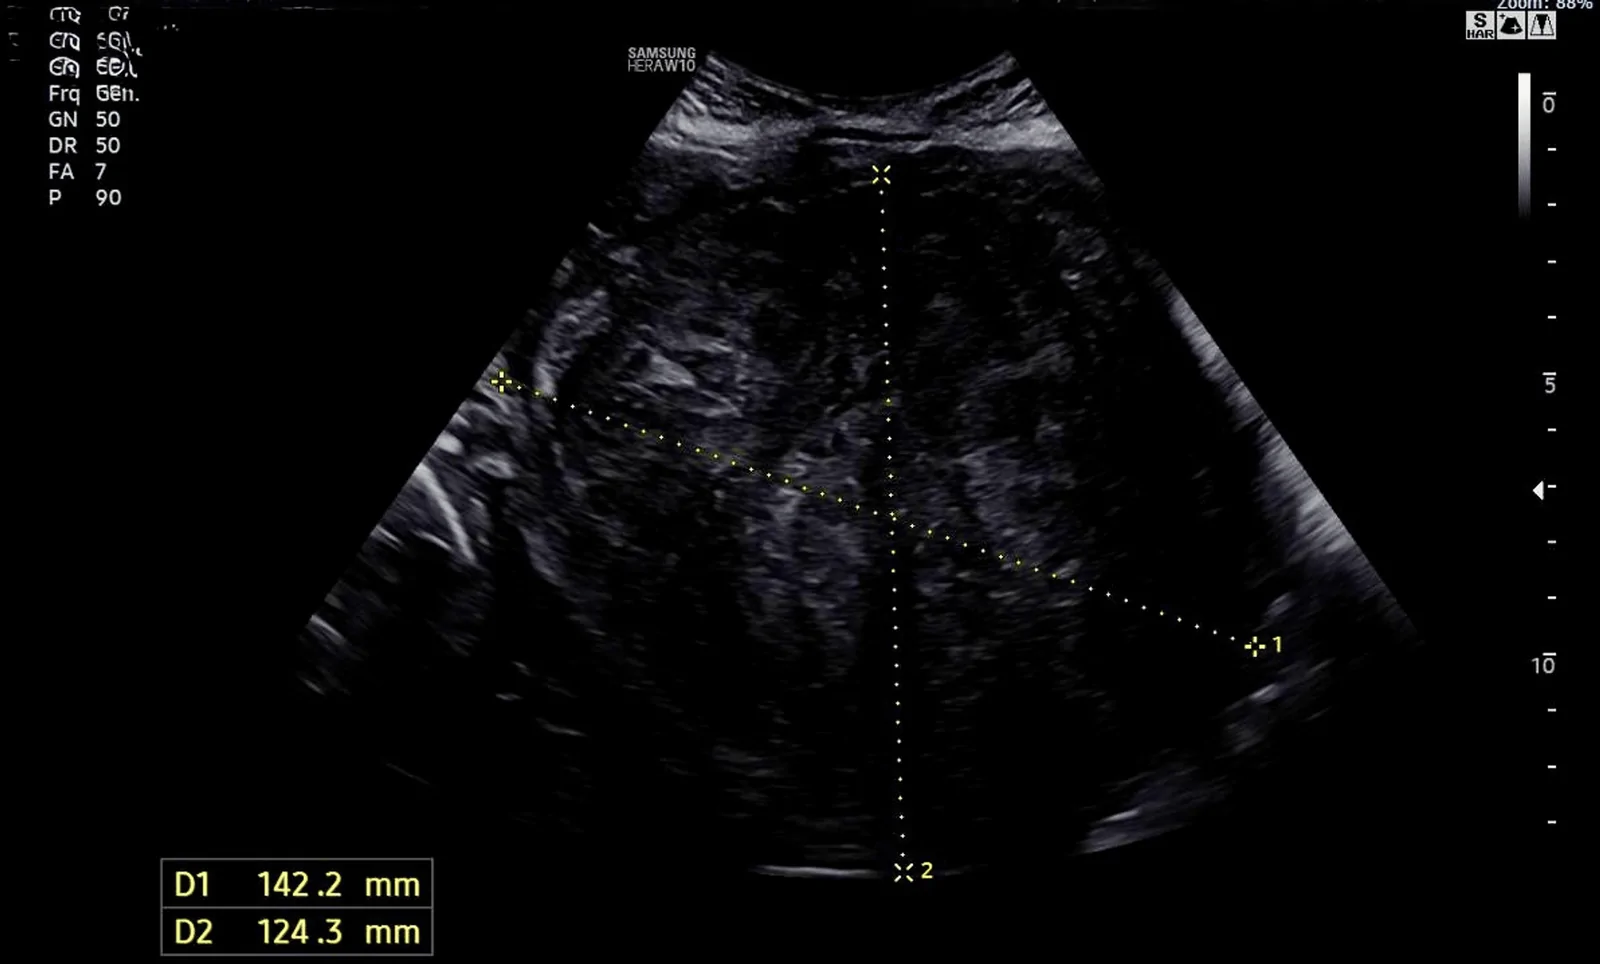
Anomaly scan ultrasound demonstrating a large uterine fibroid measuring approximately 142.2 × 124.3 mm.
